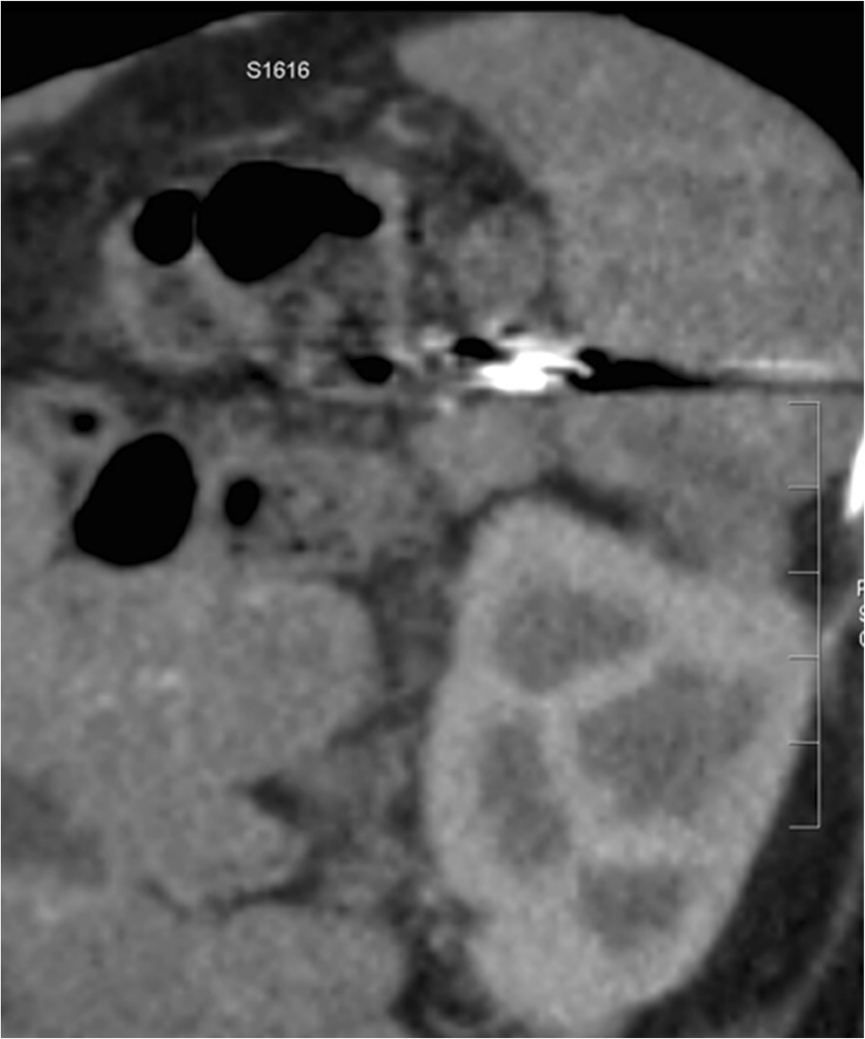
Computed tomography scan (coronal view) performed 3 months after the second procedure confirmed the complete exclusion of the splenic artery aneurysm.
